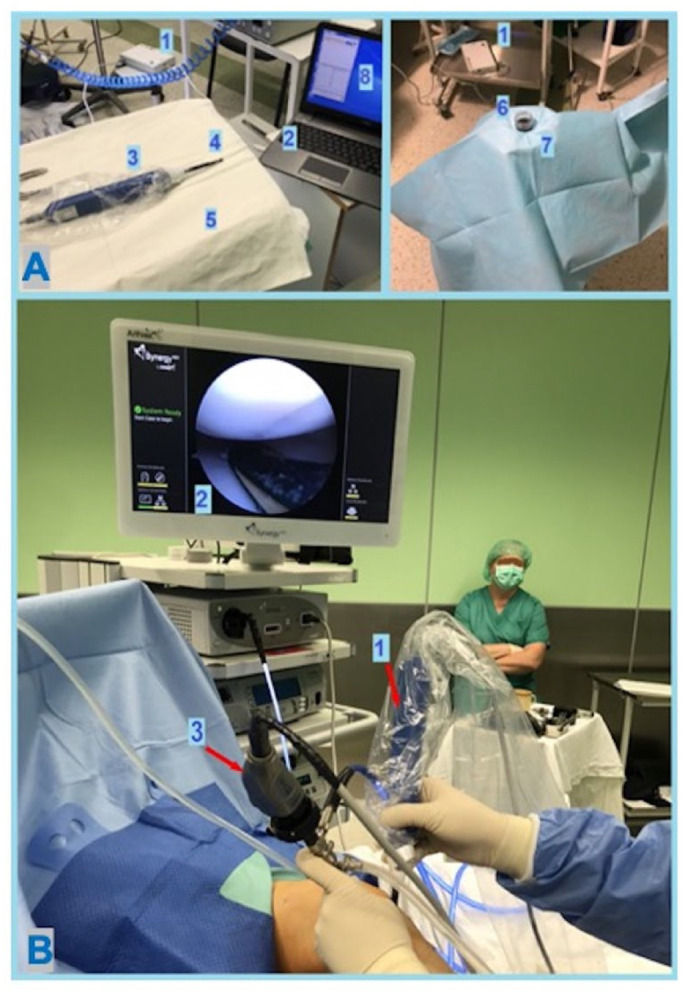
Electromechanical evaluation during knee arthroscopic surgery. (A) Preparation for electromechanical measurements. (1) Arthro-BST electrical isolation box. (2) Laptop computer. (3) Arthro-BST handle. (4) Sterile tip with electrodes. (5) Sterile table. (6) Sterile container with saline. (7) Sterile-covered ultrasound bath. (8) Arthro-BST software. (B) Electromechanical assessment during arthroscopy. (1) Sterile draped Arthro-BST handle with attached tip. (2) Sterile tip with electrodes at the measurement site of medial femoral condyle weight-bearing surface. (3) Regular arthroscopic camera.
